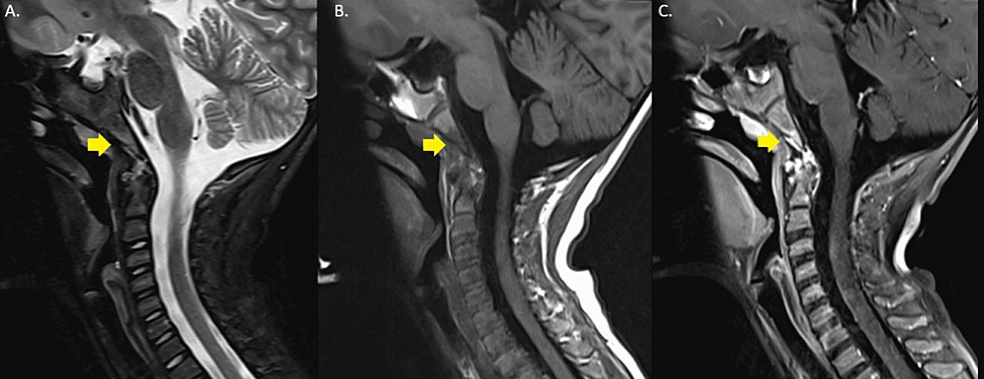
Skull base osteomyelitis (A) Sagittal section T2-weighted image with fat supression of the cervical spine; (B) Sagittal section T1-weighted image pre-contrast of the cervical spine; (C) Sagittal section T1-weighted image post-contrast of the cervical spine.
